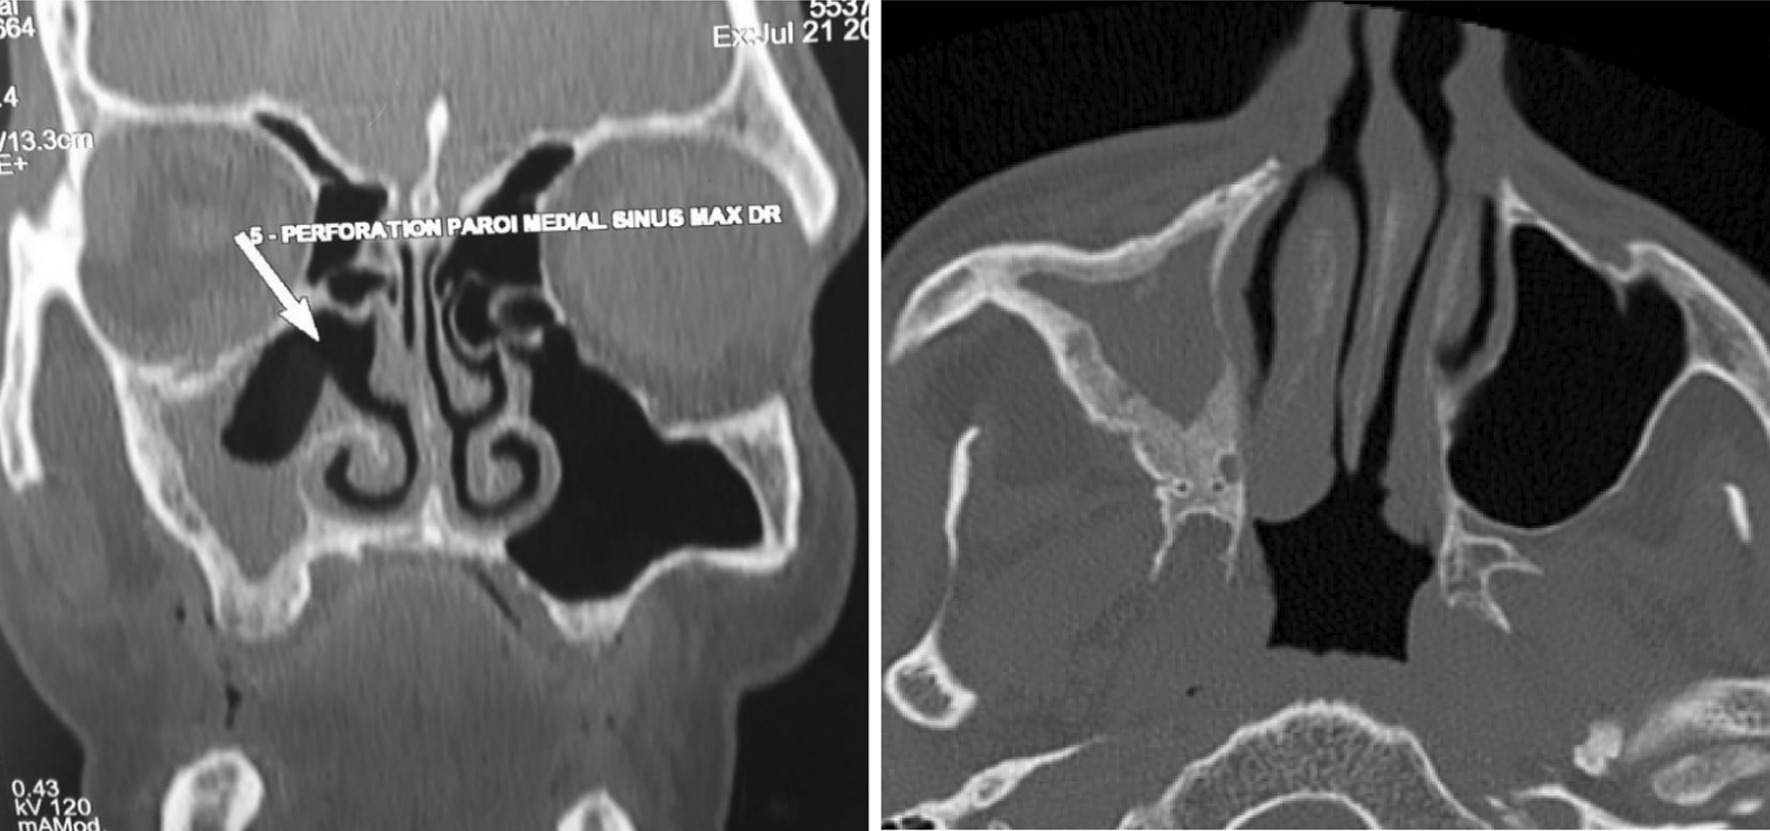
Sinus CT scan, axial section, frontal section: filling of the right maxillary sinus (sinusitis) with an erosion of the bone wall
